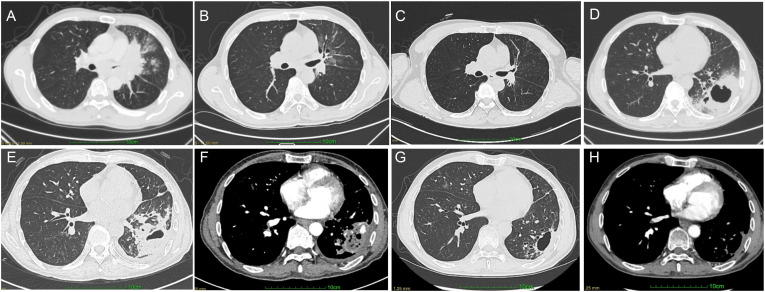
(A–H) Serial chest CT images showing evolution of the left hilar tumor and the left lower infectious lesion.
